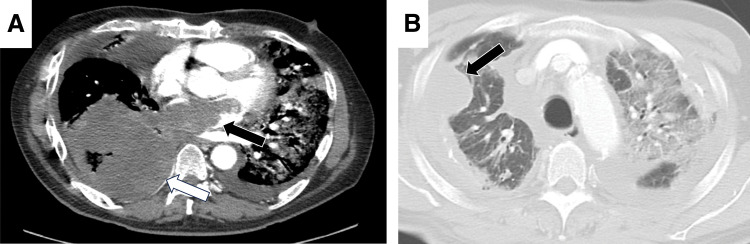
(A) Preoperative contrast-enhanced CT reveals a large mass in the right lung field (white arrow) and a lesion filling the left atrium (black arrow). (B) Preoperative CT using lung window setting demonstrates a wedge-shaped mass in the right upper lung field (black arrow) in addition to the pulmonary lesion contiguous with the left atrium.
